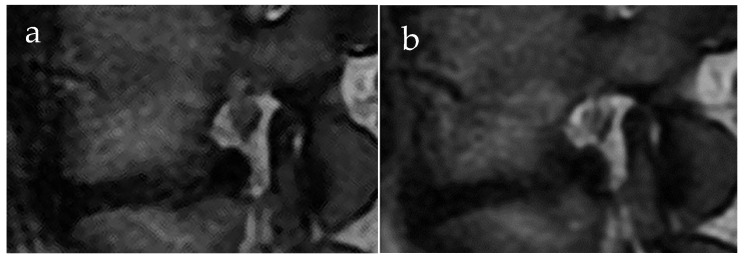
MRI of a left L4–L5 foramina acquired without loading of the spine (a) and with loading (b). A change in the foraminal shape and increase in disc bulging can be observed; the nerve root is surrounded by fat in both MR images.
